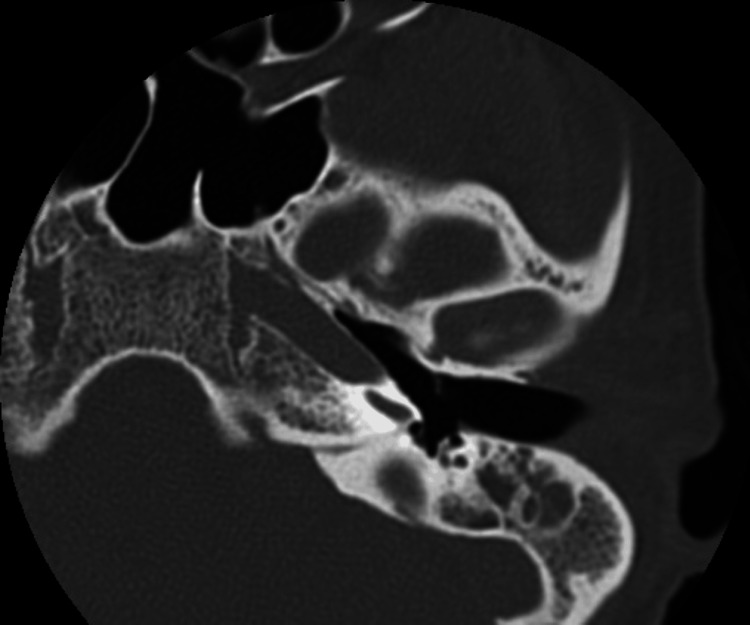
Sample error image of a misclassified SCOM CT scan image using the Random Forest model SCOM: suppurative chronic otitis media; CT: computed tomography.
